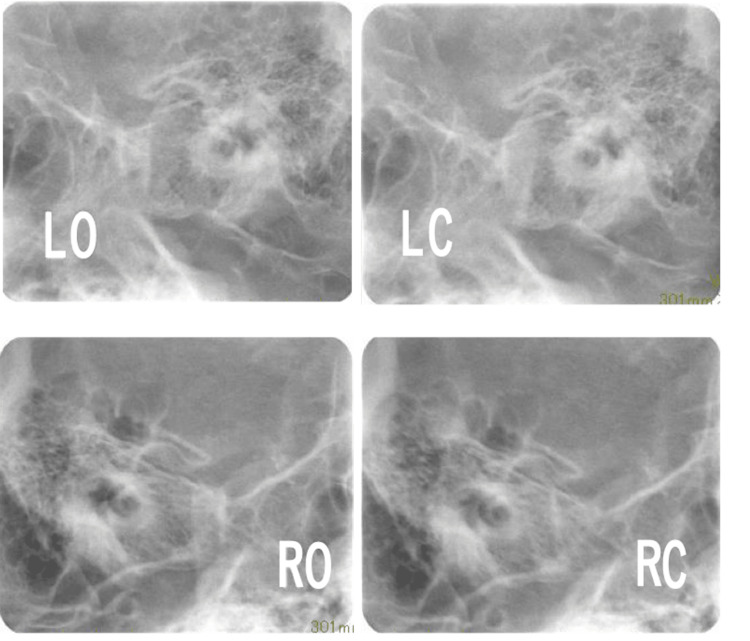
Pretreatment evaluation. Schuller’s radiographs. LO, left open; LC, left close; RO, right open; RC, right close.
